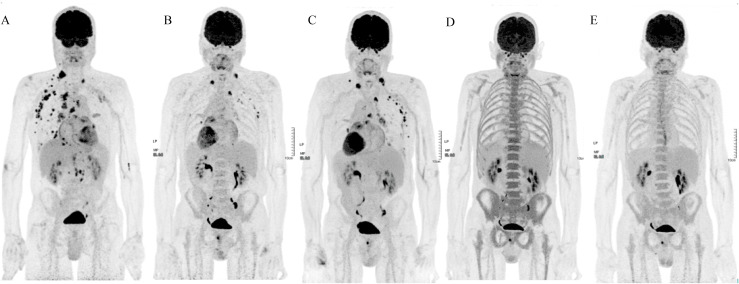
FDG-PET/CT findings in Case 1 (HER2-mutated metastatic non-small lung cancer) during the course of treatment. (A) Baseline FDG-PET/CT before second-line therapy showing hypermetabolic involvement of supradiaphragmatic and infradiaphragmatic lymph nodes, pleural lesions, and right lung parenchymal progression (MIP). (B) Partial metabolic response (PMR) under docetaxel–trastuzumab–pertuzumab, with decreased uptake in thoracic and abdominal disease sites (MIP). (C) Metabolic progression under trastuzumab–pertuzumab maintenance, with increased activity in thoracic lesions and new distant metastases (MIP). (D) Early PMR after initiation of trastuzumab-deruxtecan (T-DXd), with marked reduction of FDG uptake and disappearance of previously identified intracranial disease (MIP). (E) Complete metabolic response (CMR) on FDG-PET/CT, with full resolution of all previously hypermetabolic lesions (MIP).
